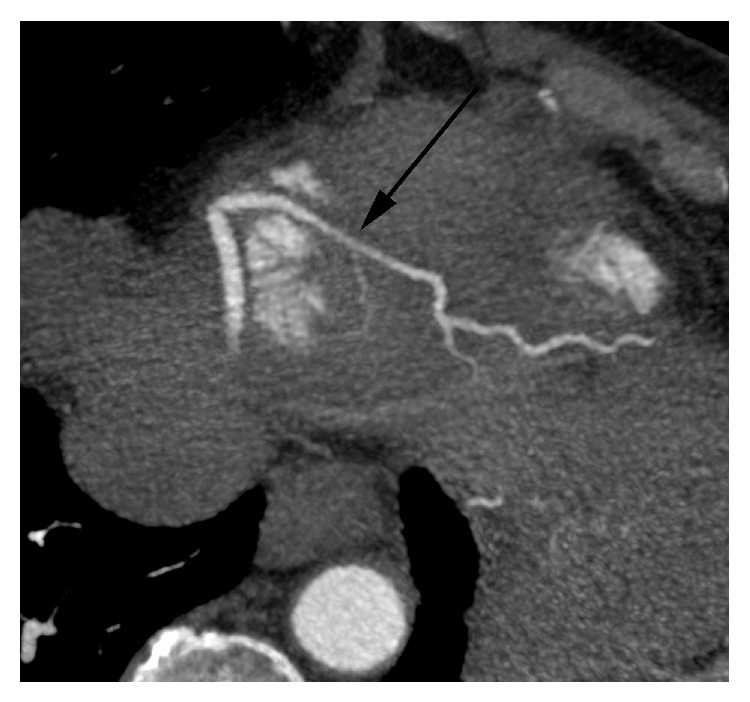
4.5 mm maximum intensity projection curved multiplanar reformatted cardiac CT image demonstrating smooth narrowing of proximal/mid portion of early arising PDA with no discernable plaque.
